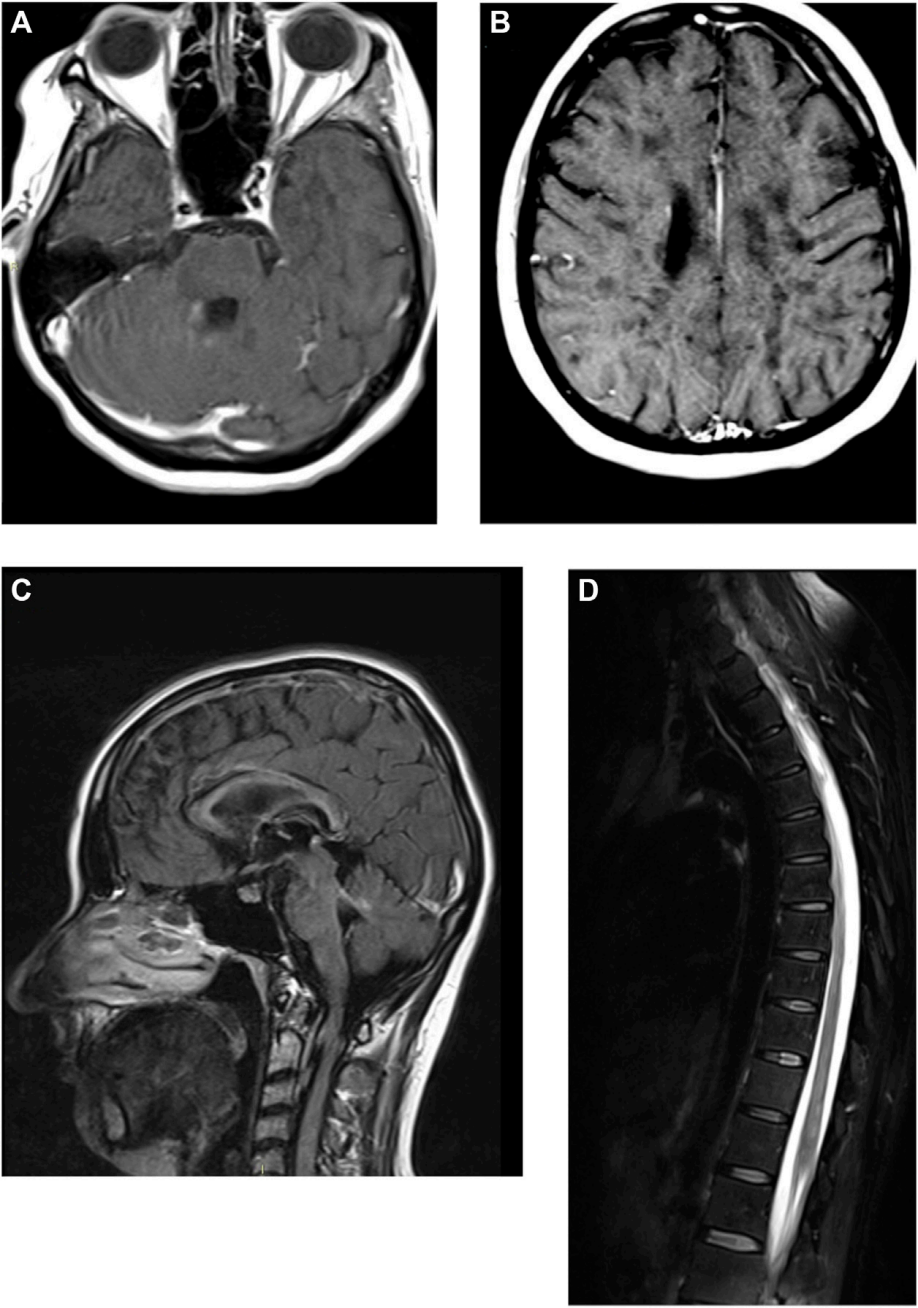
Brain and spine magnetic resonance imaging of the patient, with gadolinium enhancement. (A, B) T1-weighted axial images of the brain. (C) FLAIR sagittal image of the brain. (D) T2-weighted image of the dorsal spinal chord.
